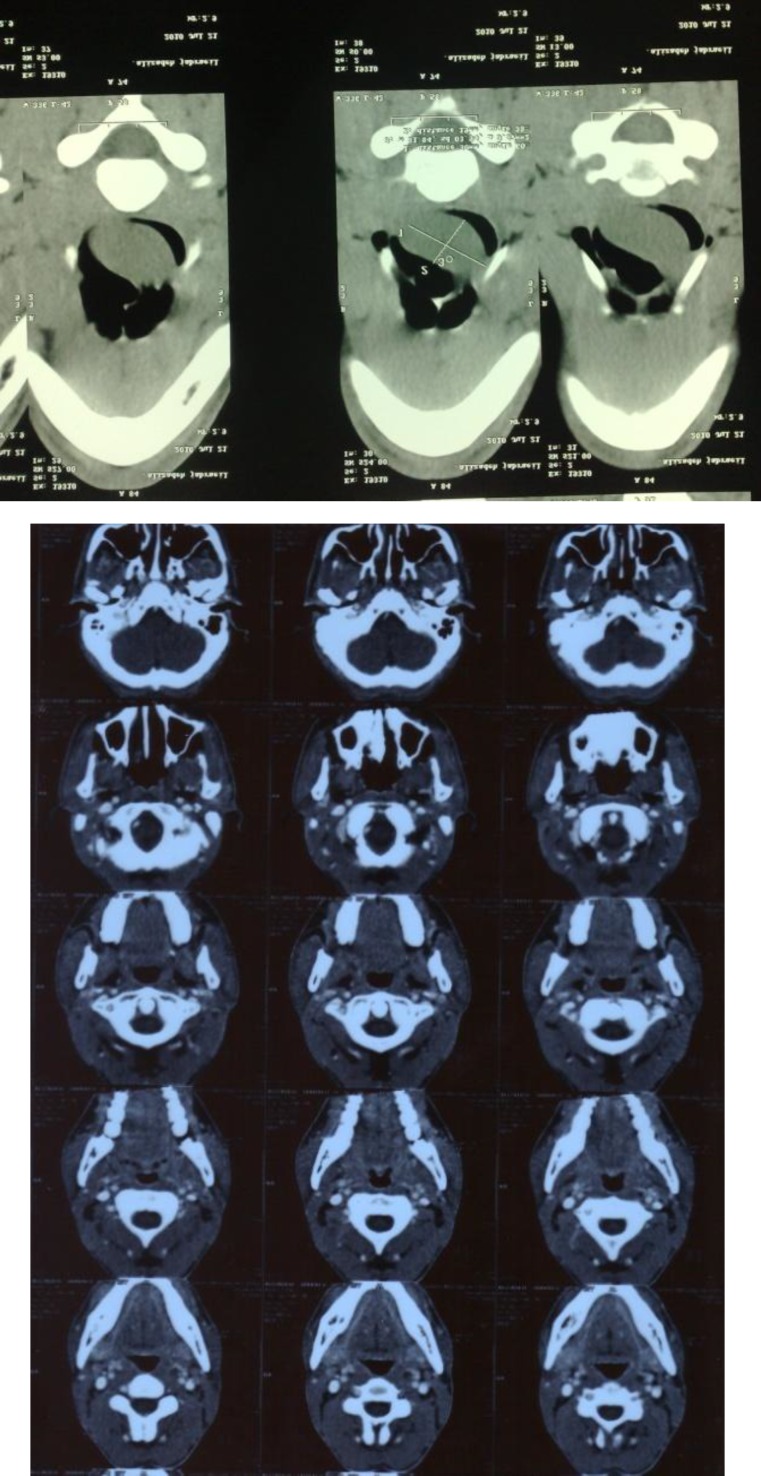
Computed tomography scan shows a solid mass, which extended from the hypo pharynx into the epiglottis and aryepiglottis fold
